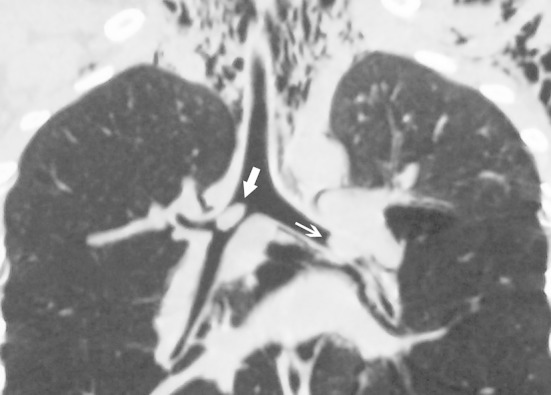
Three-dimensional reconstruction chest CT confirmed bilateral bronchial foreign body. The white arrow indicates the foreign body in the right bronchus and the black arrow indicates the foreign body in the left bronchus.
